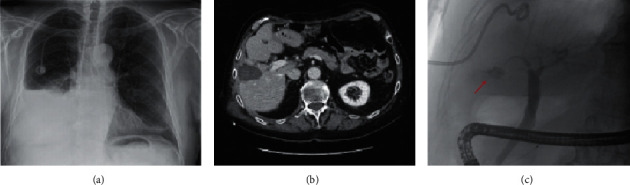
Chest plain radiograph 2 months after MWA showing a right pleural effusion (a); CT scan revealing a hypodense oval lesion of 48 × 33 mm with hyperdense core consistent with postablation liver damage (b); and ERCP demonstrating contrast leakage from right-sided bile duct (arrow) and extravasation of contrast into the right pleural cavity (C).
